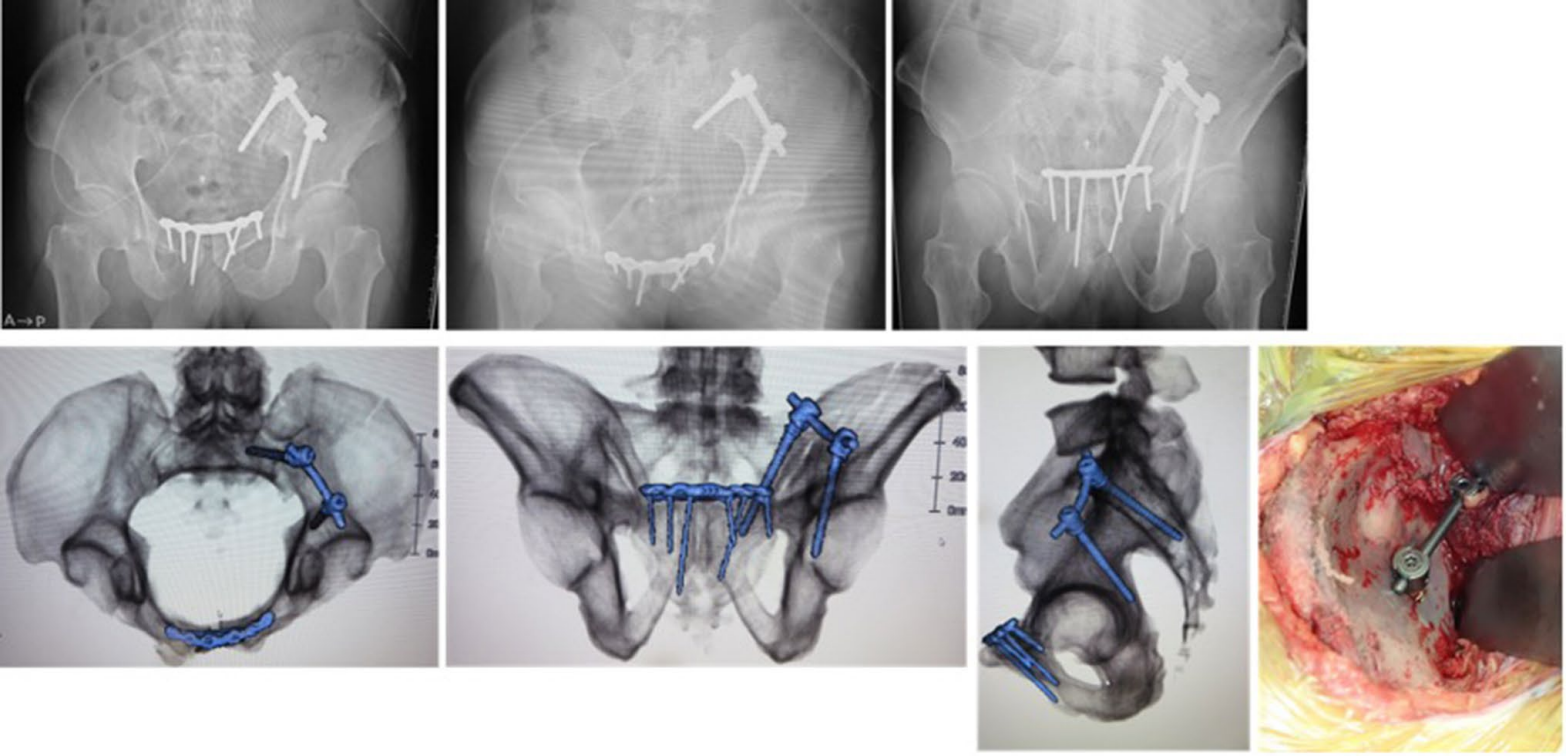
Postoperative radiographic images and a photograph of the operation. a Postoperative anteroposterior view radiograph. b Postoperative inlet view radiograph. c Postoperative outlet view radiograph. d Postoperative reconstructed inlet view 3D-computed tomography (CT) image. Screw is not outside the bone or in the sacroiliac joint. e Postoperative reconstructed outlet view 3D-CT image. Long screw has been inserted without passing through the sacral foramina or sacroiliac joint. f Postoperative reconstructed lateral view 3D-CT image. Long screw (70 mm) has been inserted in the sacrum. g A photograph of anterior sacroiliac stabilisation (different case than a–f). Surgical site is widely visualised, and the screws are bridged using a rod above the sacroiliac joint
